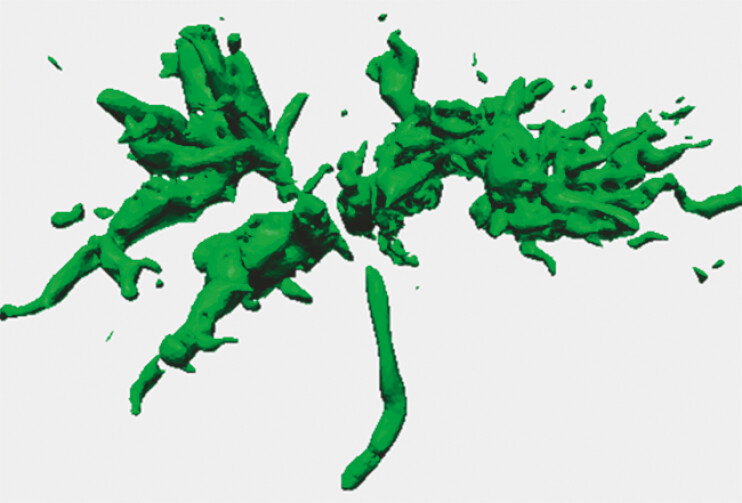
Computed tomography imaging is converted into a three-dimensional (3D) anatomical model.
